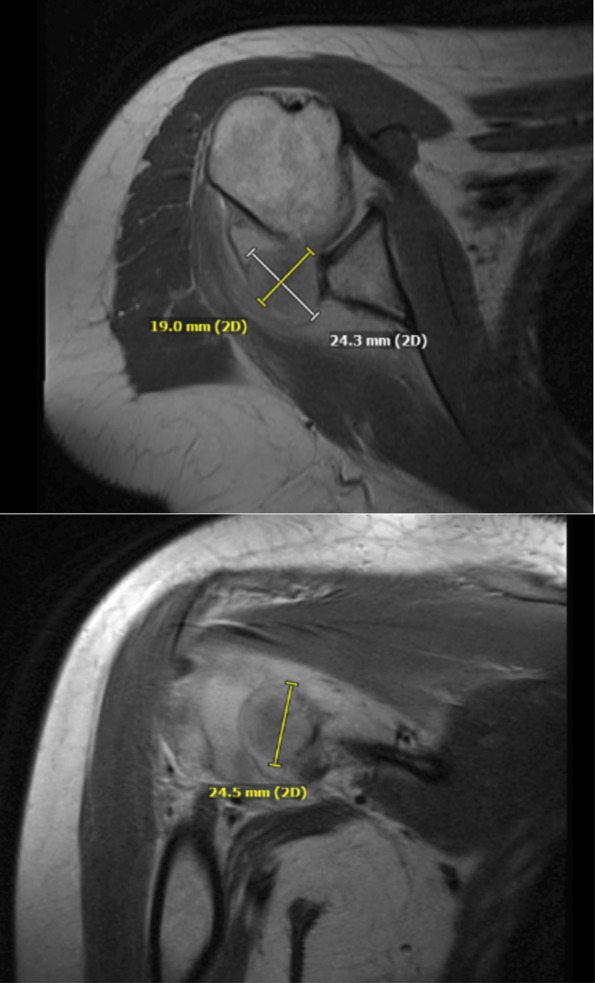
MRI showing lobulated intermediate hyperintense mass within the posterior glenohumeral recess.
